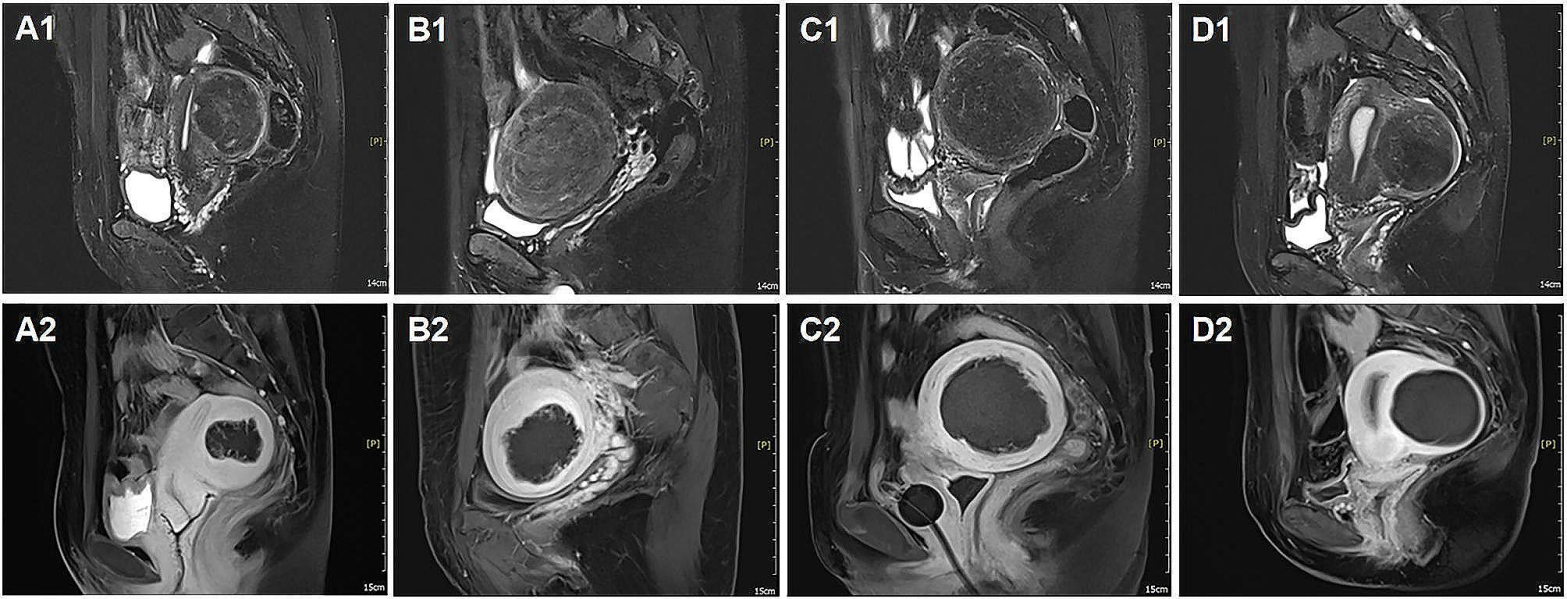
MRI of fibroids with different NPVRs. (A1–D1) T2-weighted images of fibroids before USgHIFU; (A–D2) Contrast-enhanced MR image after USgHIFU; (A2) NPVR = 30%; (B2) NPVR = 73%; (C2) NPVR = 88%; (D2) NPVR = 100%
